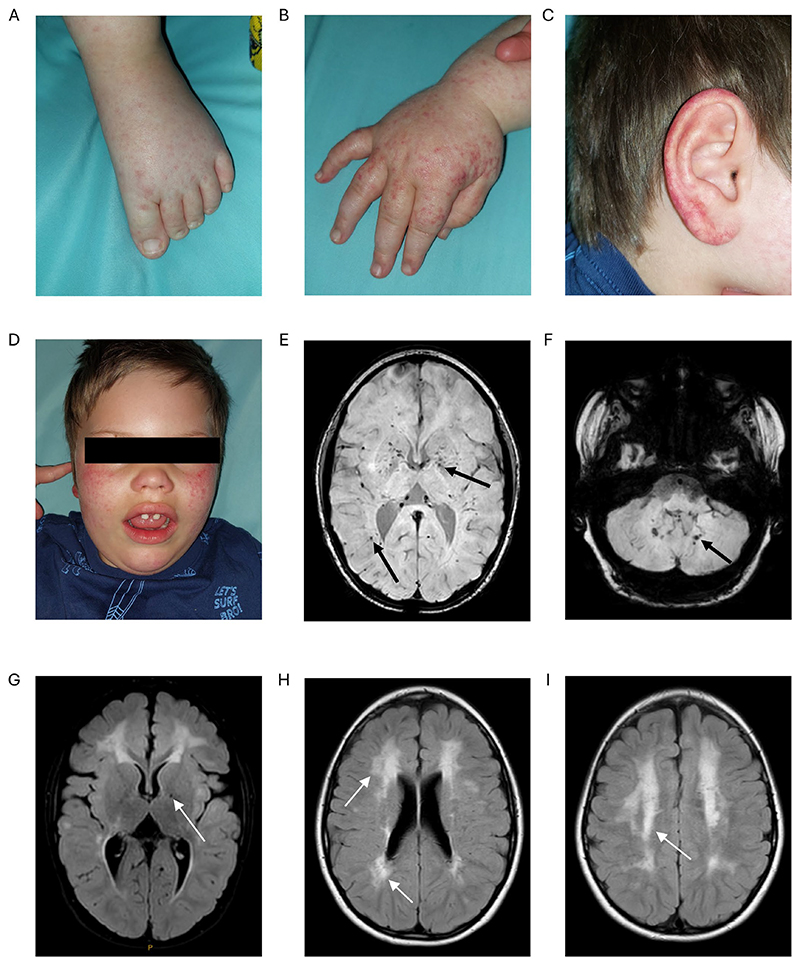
Clinical features of the AGS patient. (A-D) Vasculitic rash over the extremities, which included fingers, feet, ears, and cheeks with mild soft-tissue edema; photographs were taken at age five years, one month before baricitinib treatment started in 2022. Post-treatment photographs were not available. (E, F) Axial susceptibility weighted imaging at age 7 years, one year after baricitinib, demonstrating multifocal, symmetrical areas of blooming artifact in the basal ganglia, and deep white matter of the cerebral hemispheres (E) and dentate nuclei within the cerebellum (F) (black arrows). (G-I) Axial FLAIR imaging at age 7 years demonstrating confluent symmetrical hyperintensity in the periventricular and deep white matter of both cerebral hemispheres (white arrows).
